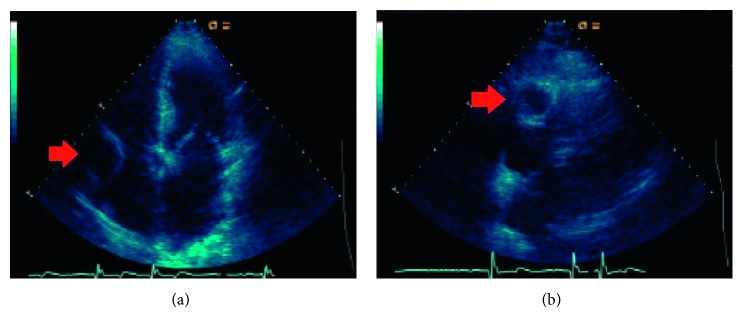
(a, b) 2D Echocardiogram showing echolucent mass in the right AV groove with mass effect. AV: atrioventricular.
